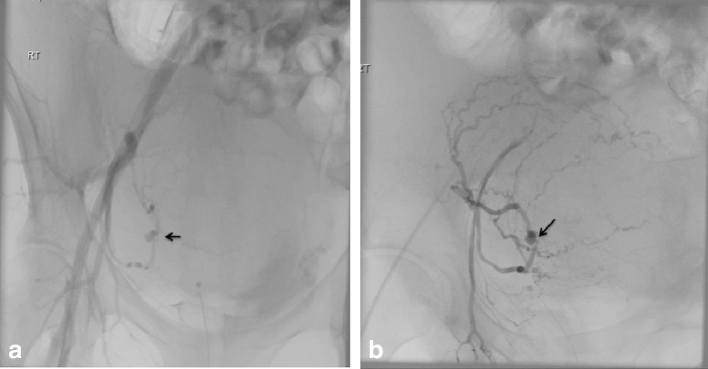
(a, b) Conventional pelvic angiogram images obtained by contrast injection via catheters placed in the right common iliac and right internal iliac artery, respectively. Both demonstrate the right uterine artery pseudoaneurysm (arrows) as seen on the CT angiogram images (Figure 2). Also shown is increased vascularity of the postpartum uterus.
